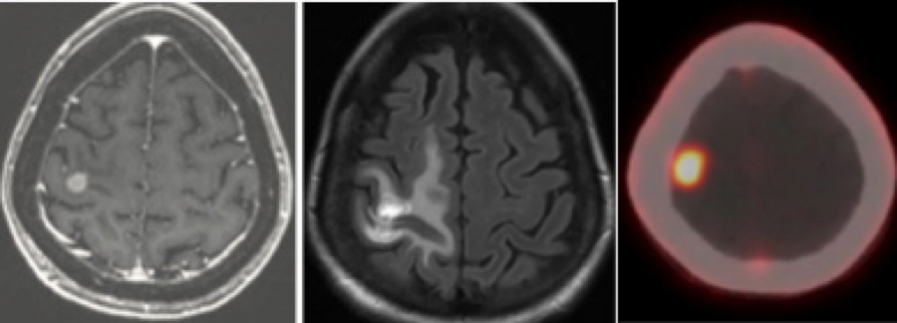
Pre-radiosurgery Images Pre-radiosurgery T1 post-gadolinium MRI demonstrating a 1 cm metastasis in the right motor strip, adjacent to the right-hand motor area (left). At six-month follow-up, there was significant post-radiosurgery fluid-attenuated inversion recovery (FLAIR) signal (middle), which demonstrated significant fludeoxyglucose (FDG) uptake on PET-CT (right).
